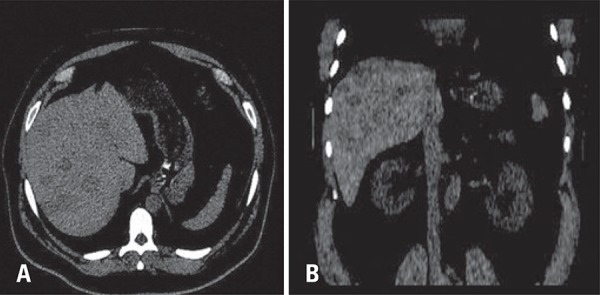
(A) Non-enhanced computed tomography on axial A coronal reformatted. (B) Showing multiple hypoattenuating liver nodules
